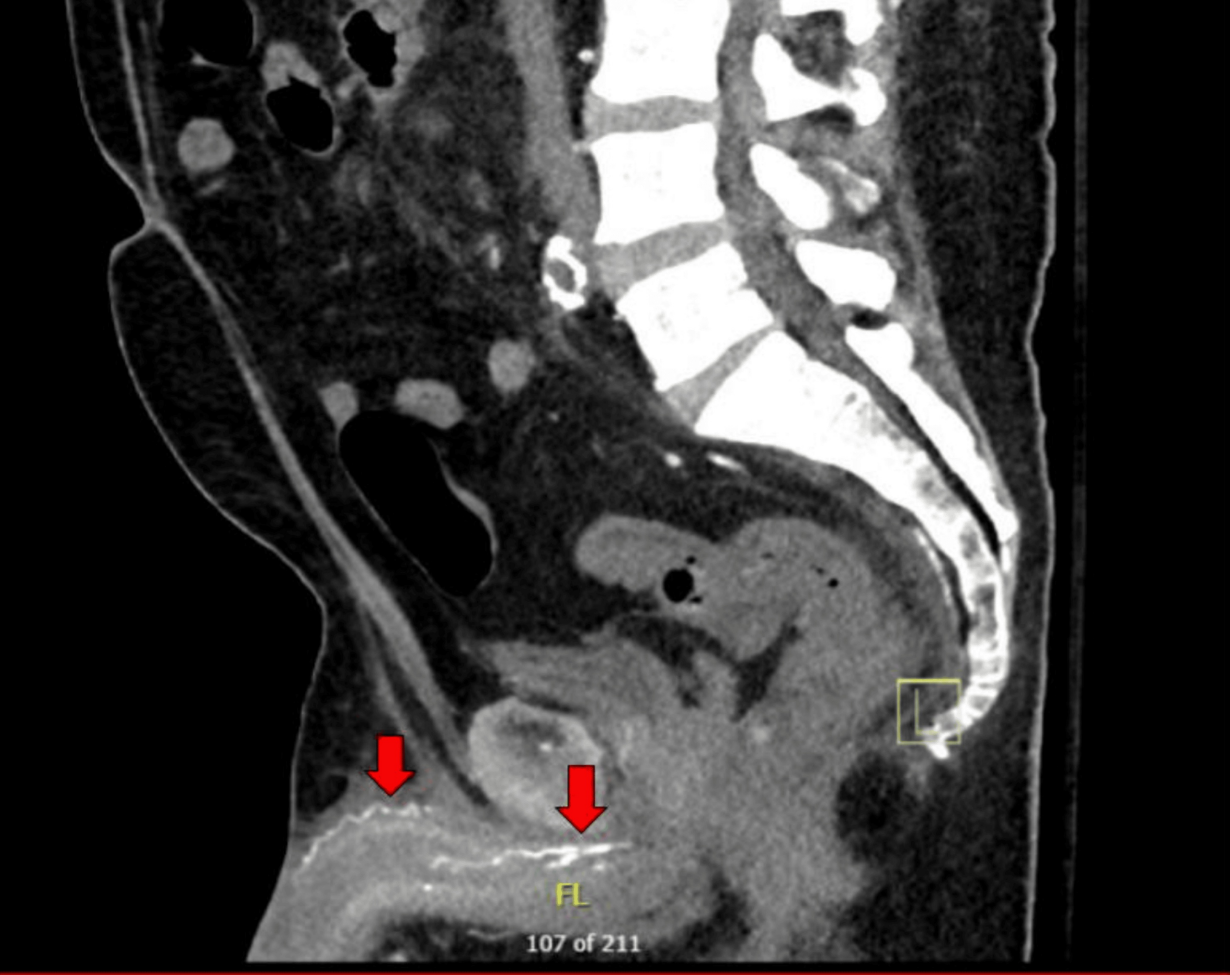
CT abdomen pelvis without IV contrast Sagittal plane delineating extensive calcification in the cavernosal arteries (red arrows)
